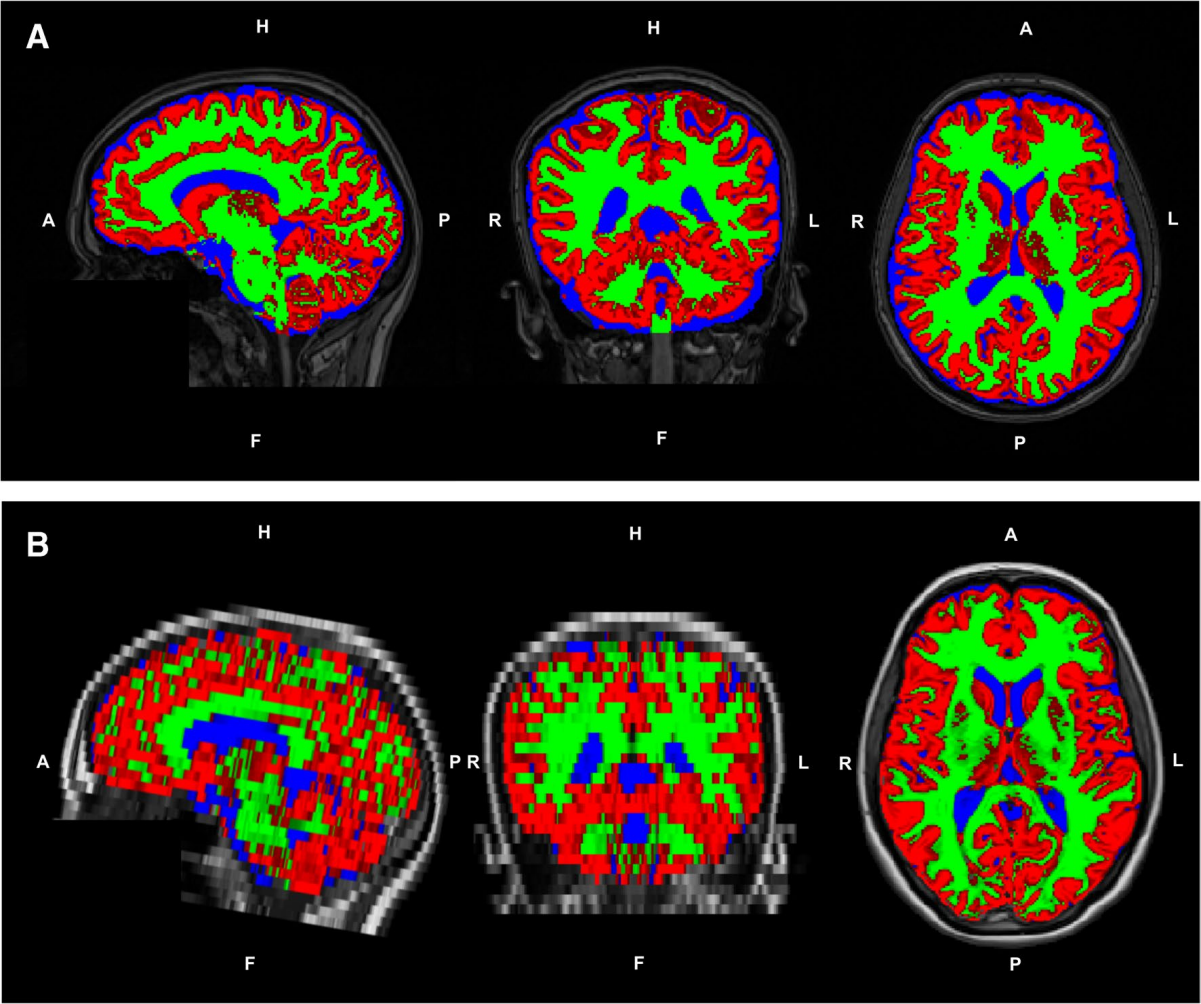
Comparison of tissue segmentation maps of a a healthy participant (female, 36 years old)﻿ generated by SIENAX (A) and SyMRI (B) shown on corresponding T1-weighted images; green overlay: white matter; red overlay: grey matter; blue overlay: cerebrospinal fluid
